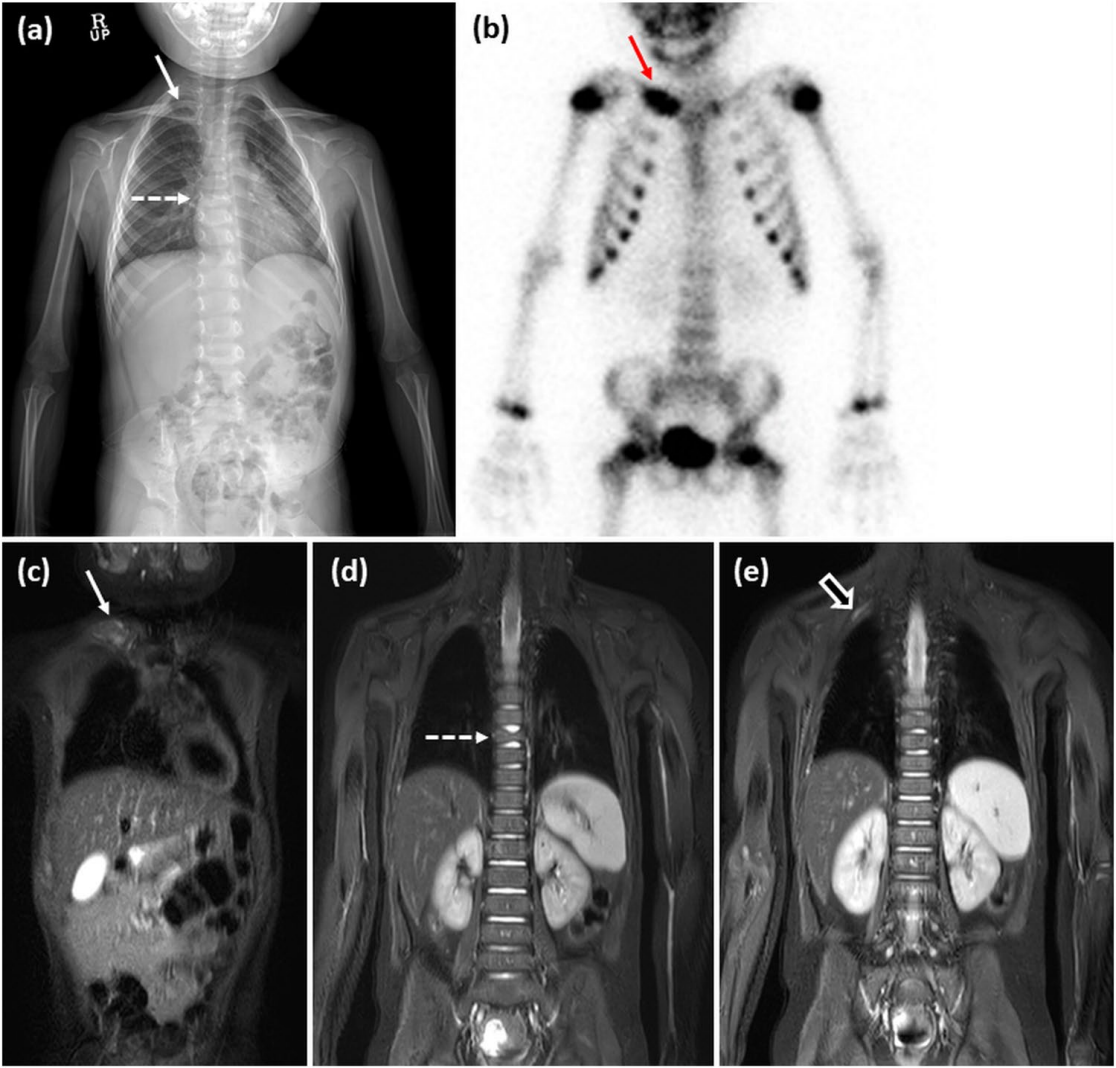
Skeletal survey (a), bone scan (b) and STIR coronal images of WB-MRI (c–e) of a 6-year-old boy with a pathologically-confirmed LCH lesion in the right clavicle. The lesion in the clavicle (solid arrow) was detected in all three imaging modalities (a–c); however, the lesion in the T8 vertebral body, appearing as a partially collapsed vertebral body, was noted only on skeletal survey (a) and WB-MRI (d), and the lesion in right first rib was noted only on WB-MRI (e). Although an additional lesion in the right first rib was detected on WB-MRI but not by the skeletal survey, the risk stratifications by WB-MRI and skeletal survey were identical for single system LCH with multiple bone lesions or CNS risk lesions.
